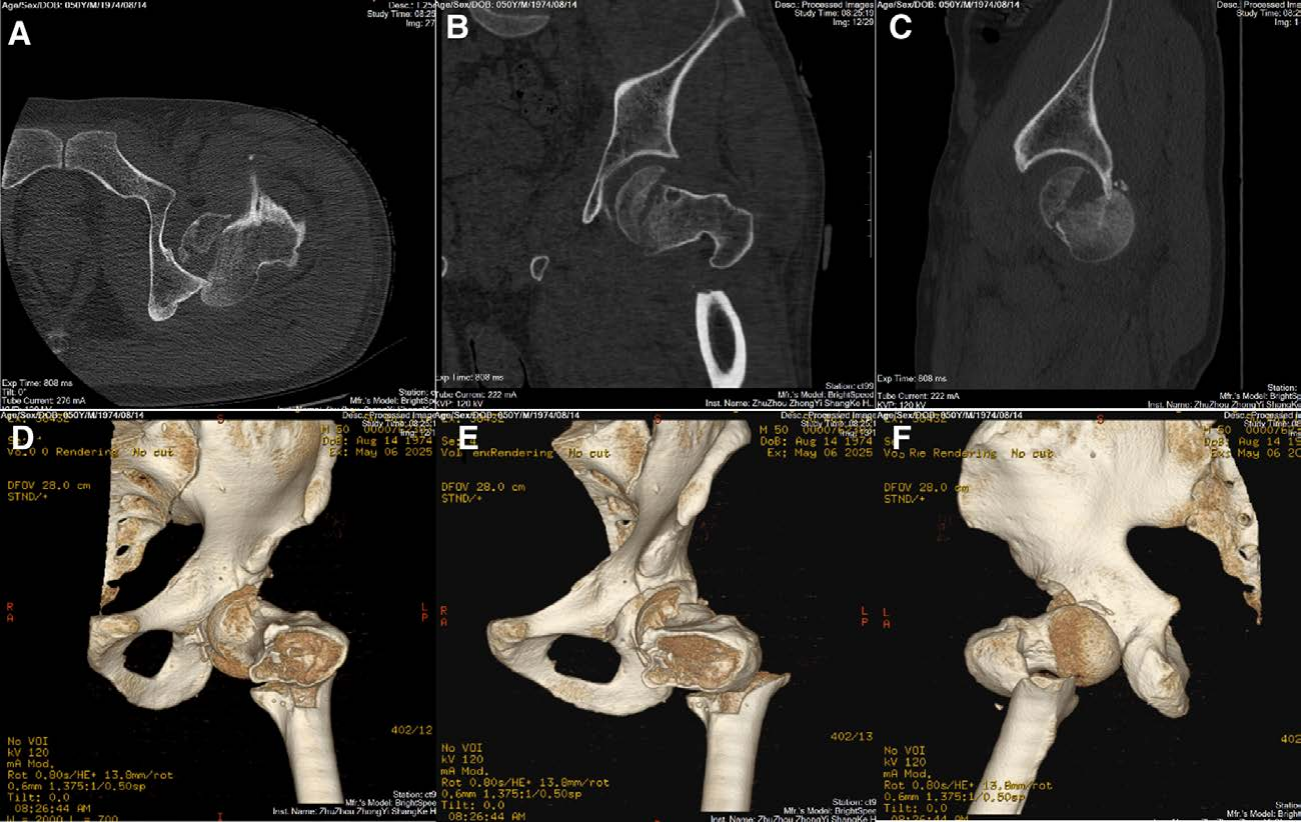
Computed tomography images in horizontal (A), coronal (B), and sagittal (C) planes along with 3D reconstruction of the left hip. A separate FHF fragment remained within the acetabulum (visible in A and C). Imaging revealed a 2-part intertrochanteric fracture with clear bisection of the lesser trochanter (visible in D, E and F).
